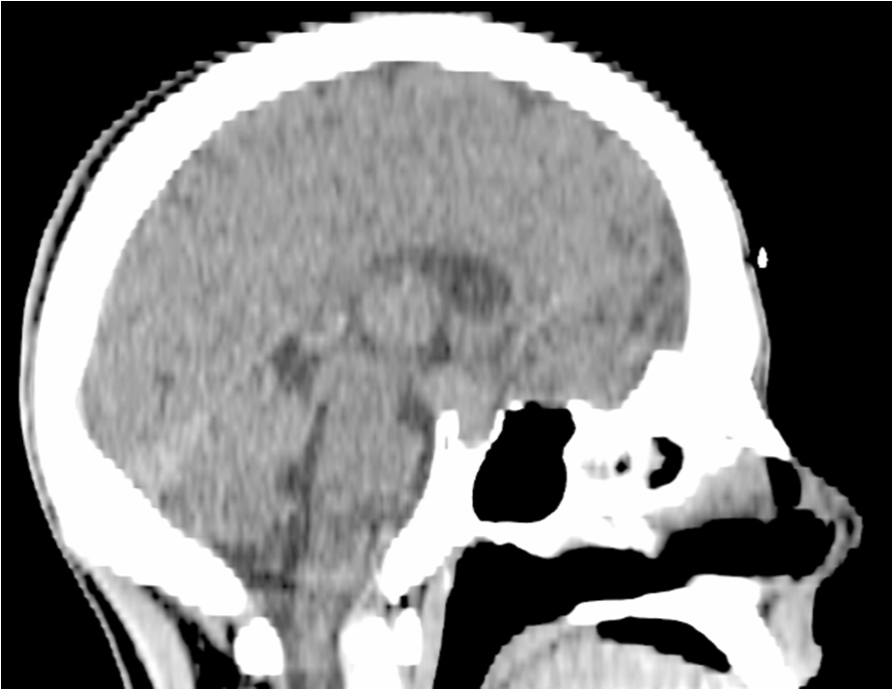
Sagittal computed tomography scan showing a pituitary mass.
